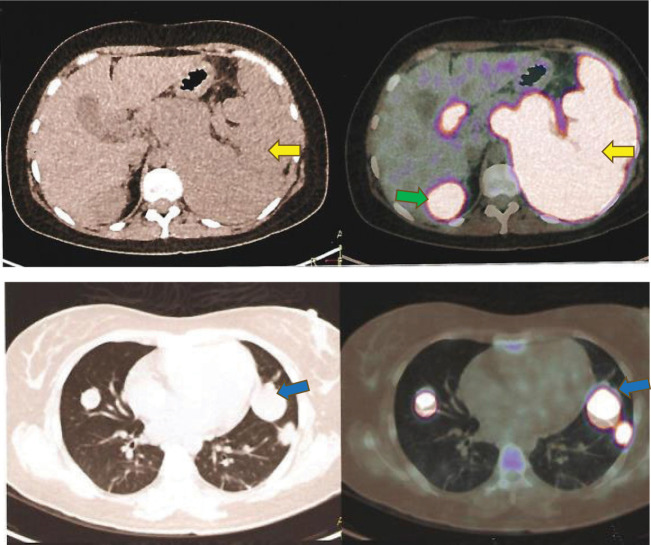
(A) Chest Xray before 3rd line Chemotherapy showing bilateral lung metastasis (green and yellow arrows). (B) After 3 cycles of 3rd line Chemotherapy, right lung metastasis size was reduced (green arrow) in comparison to left lung (yellow arrow). (C) After 3 cycles of 3rd line chemotherapy CT scan of abdomen shows stable response of disease primary tumor (Yellow Arrow), liver metastasis (Green Arrow) in comparison to PET CT before 3rd line chemotherapy.
